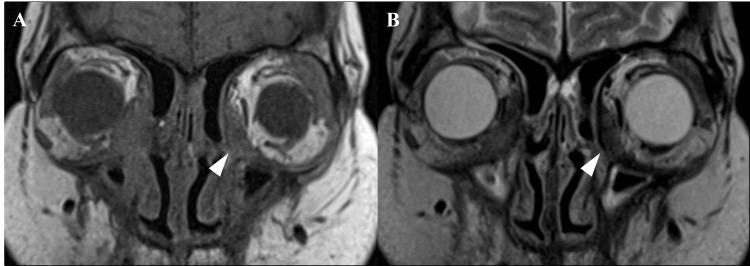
Paranasal Sinus MRI Images A: T1-weighted image showing isointense orbital mass (arrowhead) appearing to invade the medial rectus.
